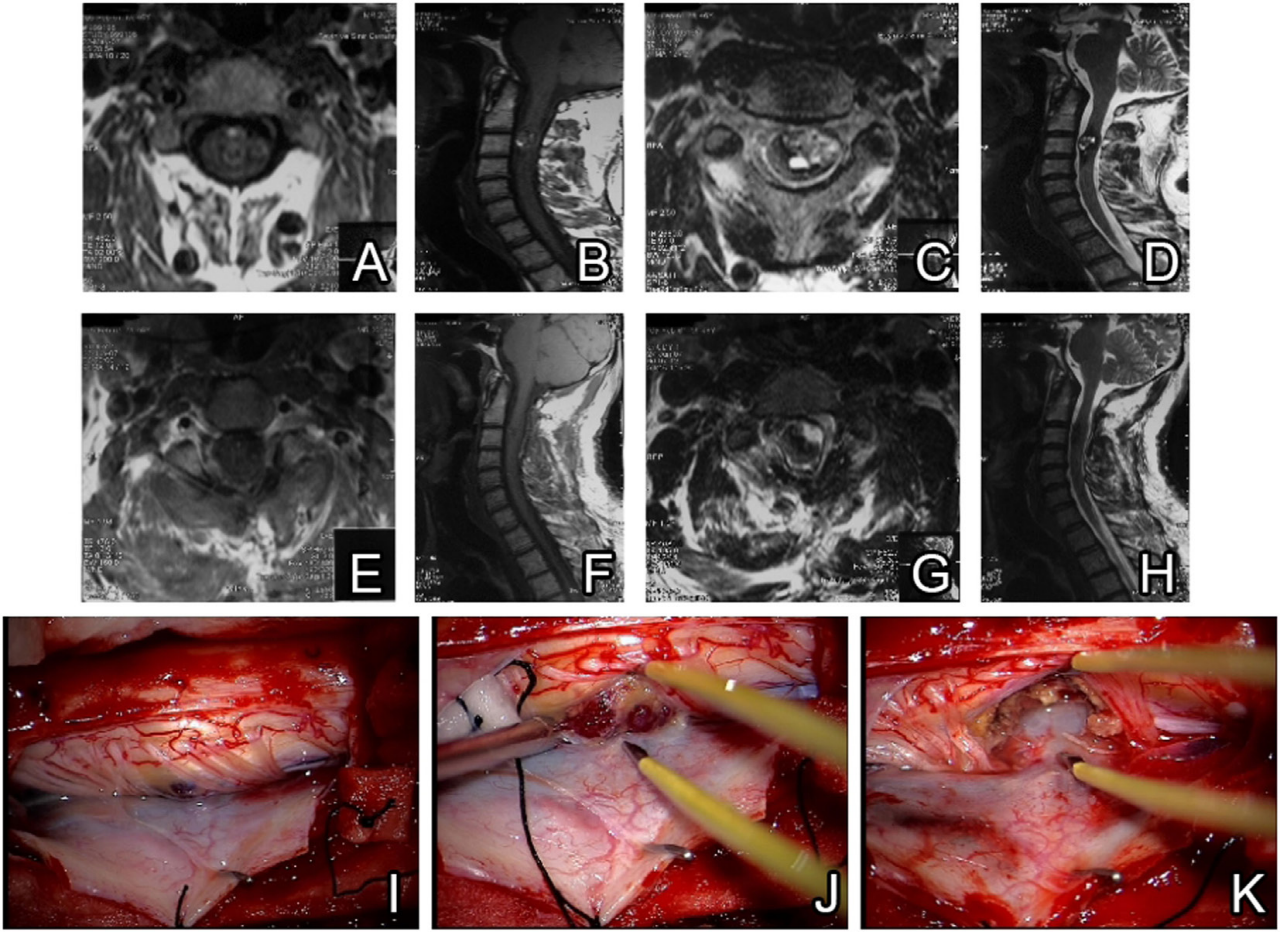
Preoperative MRI scans reveal a cervical intramedullary located cavernoma (A–D). Postoperative MRI confirms total resection without hematoma or residue (E–H). Intraoperative view of cavernoma after midline dural incision and retraction (I). Anterior intramedullary located cavernoma seen after mild retraction of spinal cord (J). After total removal of cavernoma, no residue is seen in the surgical field (K).
